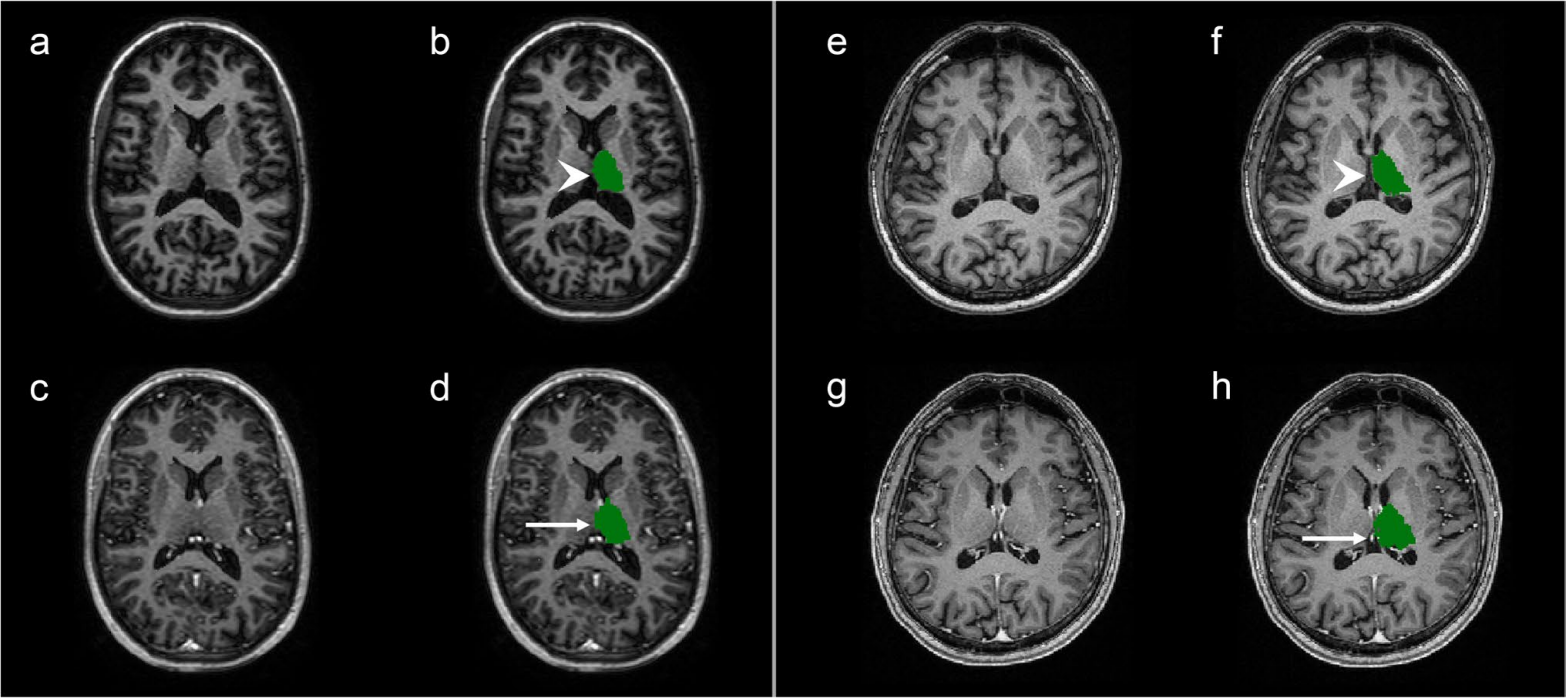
T1-weighted MRI, showing the segmentation of the left Thalamus in pre- and post-contrast images, in two different patients (subject E3 (a–d) and subject C1 (e–h)). a–d Axial slices demonstrating the typical quality of thalamus segmentations. In post-contrast images (c–d), the medial border of the left Thalamus is slightly overestimated (arrow) compared to pre-contrast images (arrowhead) (a–b), most likely due to hyperintense signal from extraparenchymal structures in the midline. e–h Axial slices demonstrating a more severe overestimation of the medial border of the left Thalamus (arrow) in post-contrast images (g–h) compared to pre-contrast images (arrow head) (e–f). Again, the segmentation of the medial border is overestimated due to inclusion of extraparenchymal hyperintense structures, in this case, the internal cerebral vein)
